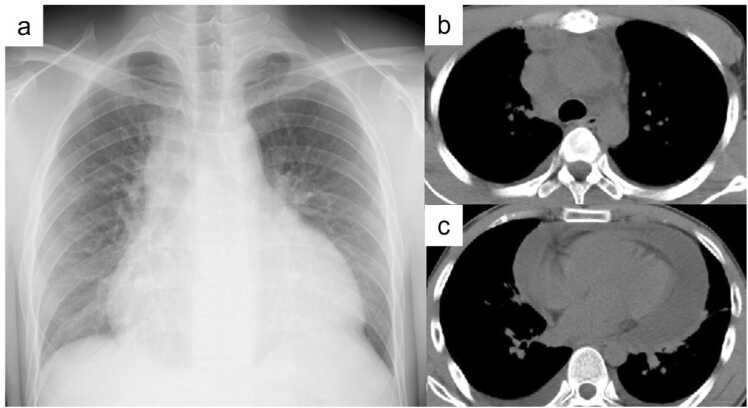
Chest X-ray after initiation of anti-tuberculosis therapy, showing improvement of mediastinal widening (a). Chest CT after three months of treatment revealing reduction of mediastinal lymphadenopathy (b) and pericardial effusion (c).
